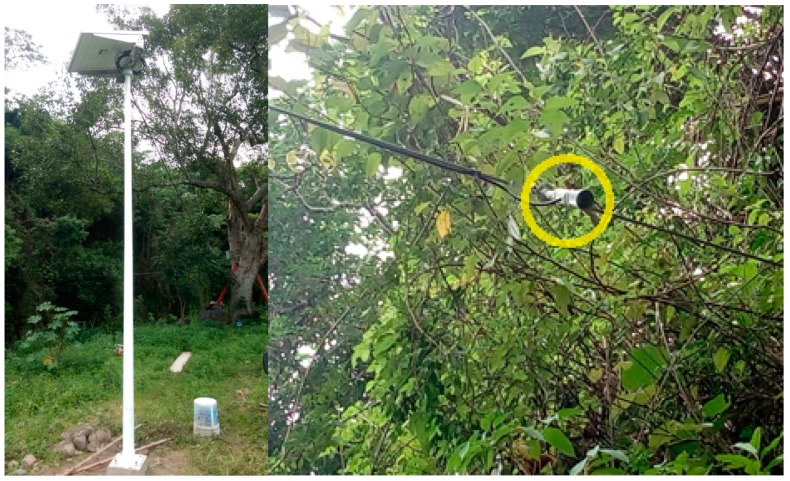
Positioned solar panel and ultrasonic sensor.
